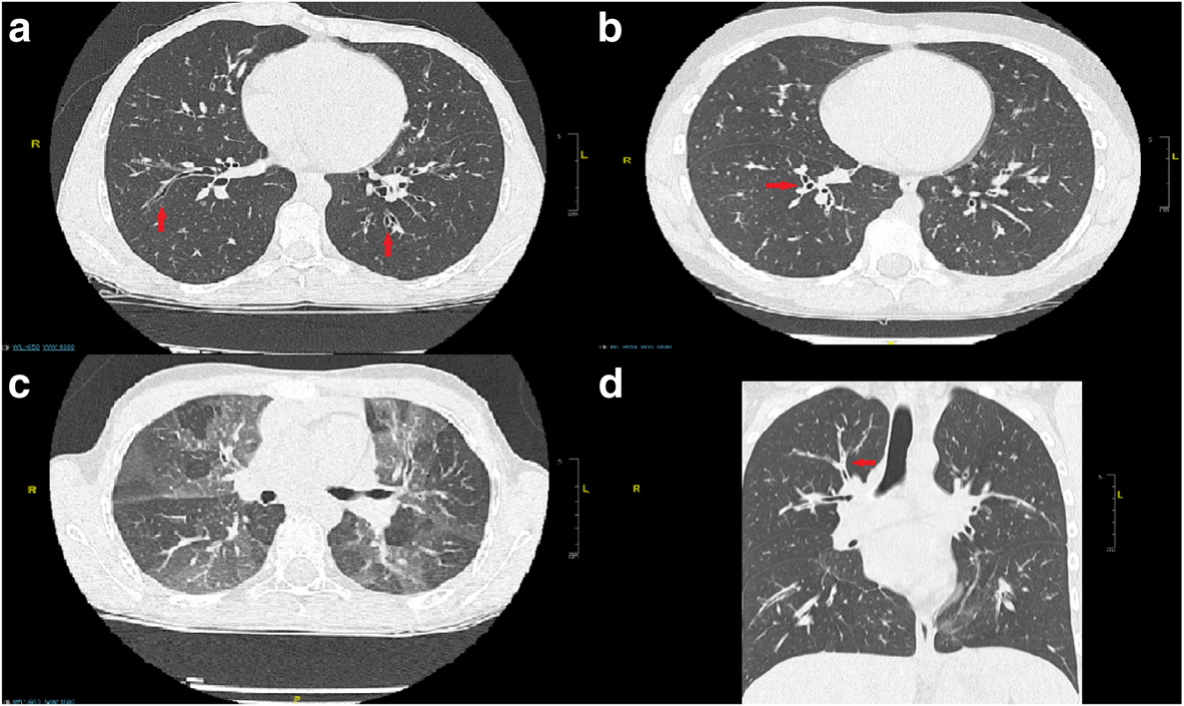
Examples of chest CT abnormalities found in our cohort of children with severe asthma: a) Bronchiectasis, b) Bronchial Wall Thickening, c) Air Trapping, and d) Mucous Plugging
